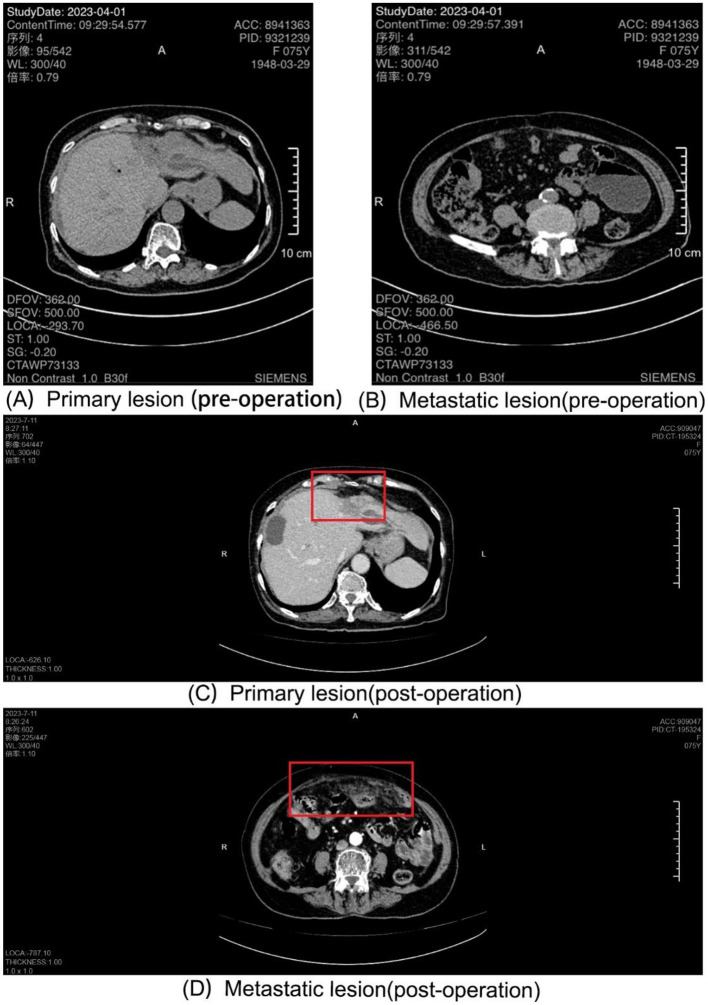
CT imaging of primary and metastatic lesions. (A) and (B) represent the primary and metastatic lesions on preoperative imaging; (C) and (D) represent those on postoperative imaging.
